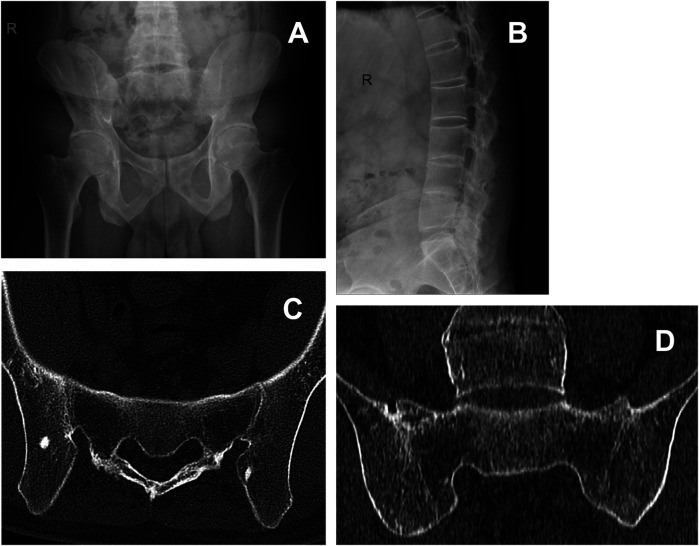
Pre-treatment radiographic assessment and two-year follow-up computed tomography (CT) in a patient with advanced ankylosing spondylitis. (A,B) Pre-treatment anteroposterior radiograph of the pelvis demonstrating characteristic advanced changes, including complete bony fusion of both sacroiliac joints with obliteration of the joint spaces, ankylosis of the lumbar facet joints, and a loss of the normal lumbar lordosis. (C) Axial CT image and (D) coronal CT image obtained two years post-acupotomy (2022). Both panels confirm the persistence of the bilateral sacroiliac joint bony fusion and sclerosis.
